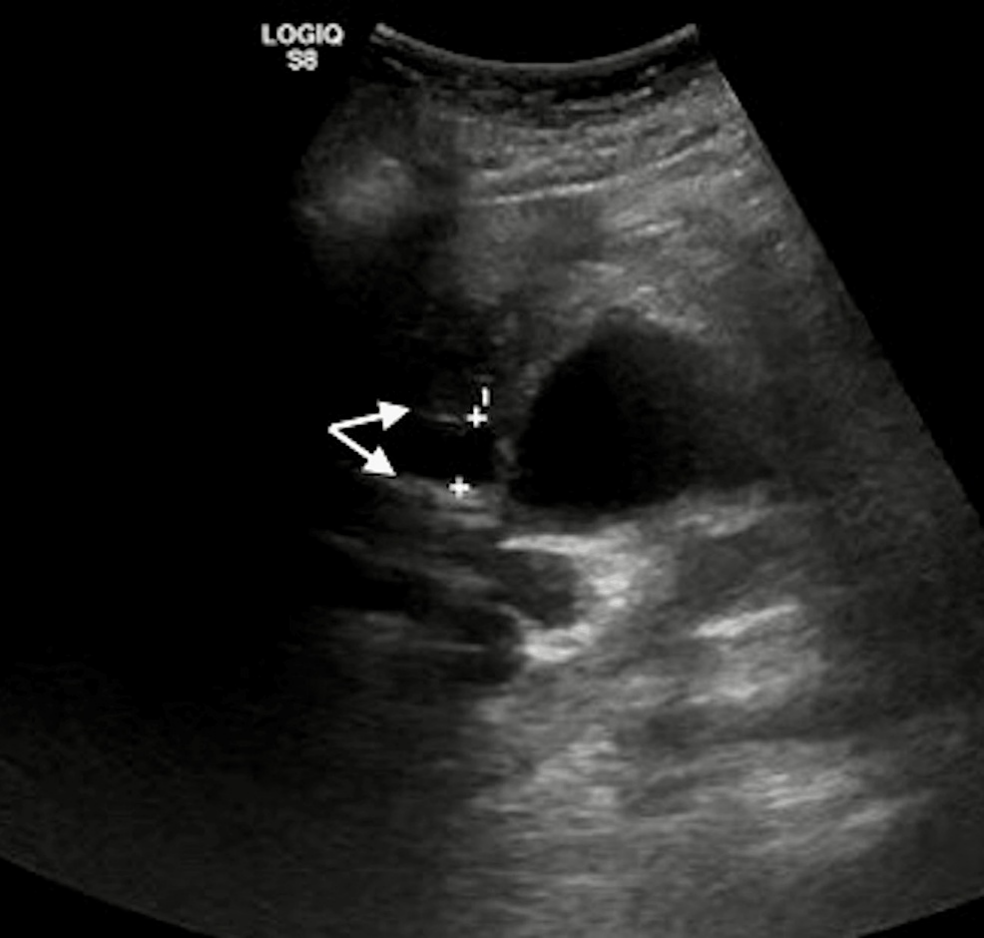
An ultrasound of the abdomen showing dilation of the common bile duct measuring approximately 1.5 cm in diameter
